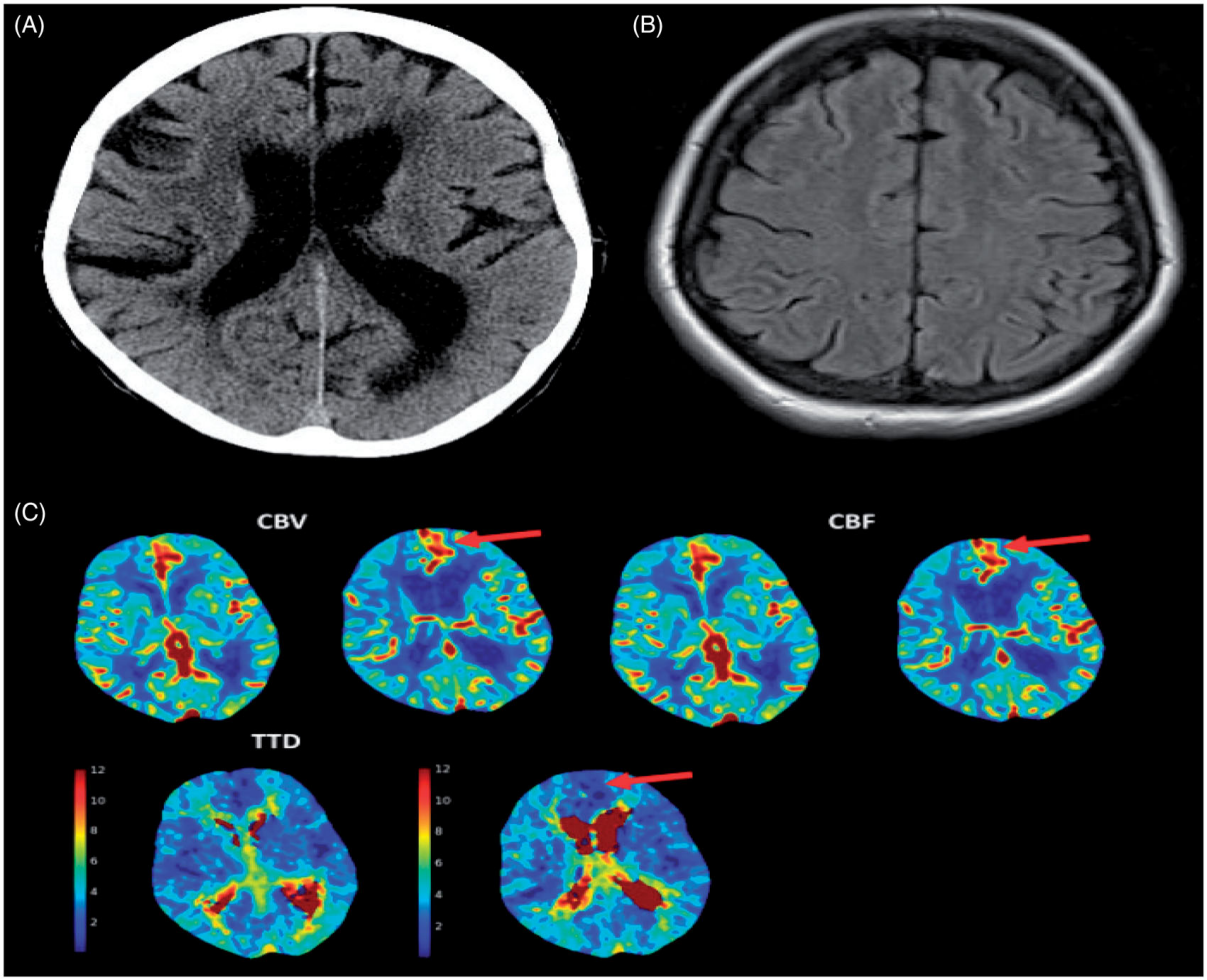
An 85-year-old female presented with expressive aphasia. A,B: Axial computed tomography (CT) head, CT angiogram (not included), magnetic resonance imaging (MRI) of the brain that also included diffusion-weighted imaging were unremarkable. The patient was discharged with the diagnosis of transient ischaemic attack (TIA). After discharge, the patient continued to have recurrent similar episodes. C: CT perfusion during one of her episodes demonstrated left frontal focal hyperperfusion characterized by increased cerebral blood flow (CBF), cerebral blood volume (CBV) and decreased time to drain (TTD). The patient was diagnosed with focal seizure with preserved awareness.
